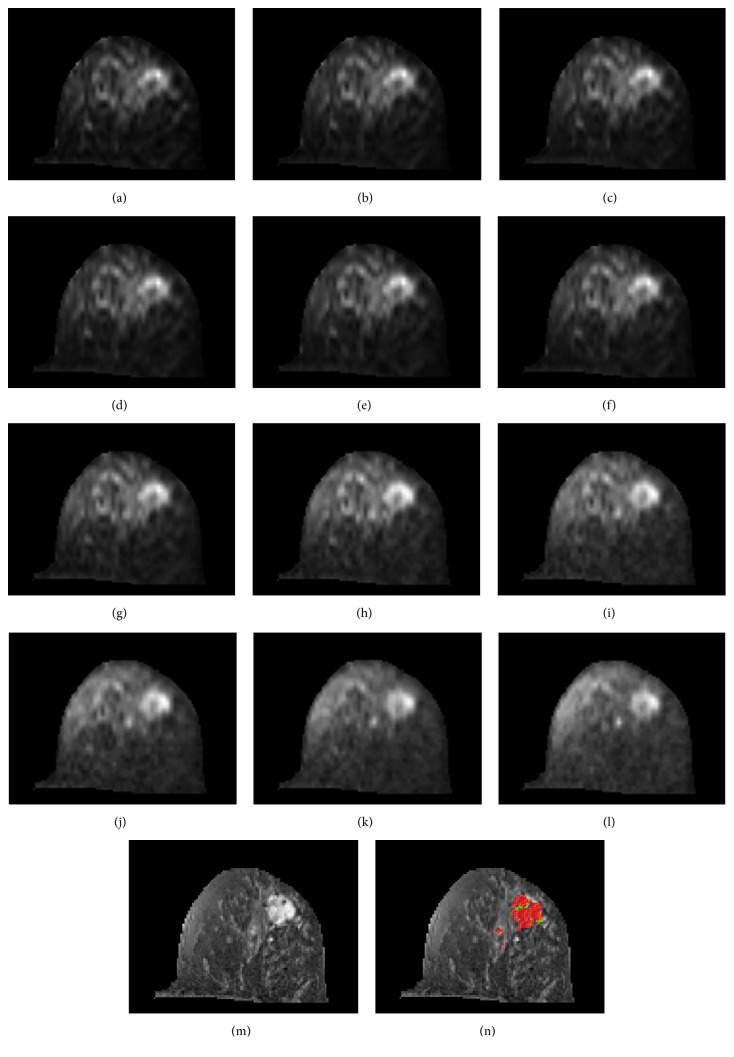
The IVIM-DWI images of left breast cancer at different* b* values: (a)* b *= 0 (b)* b *=15 (c)* b* = 30 (d)* b* = 45 (e) b = 60 (f)* b* = 100 (g)* b* = 250 (h)* b* = 400 (i)* b* = 550 (j)* b* = 700 (k)* b* = 850 (l)* b* = 1000 s/mm^2^. (m) The dynamic T1-DCE MR image. (n) Lesion mapping with red and green pixels indicating the central and peripheral tumor regions, respectively.
